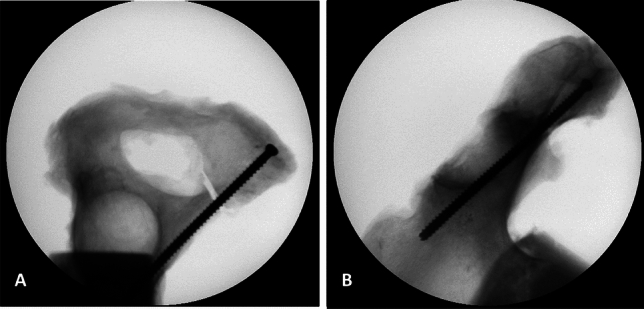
X-ray of superior ramus fracture stabilized with a 6.5 mm cannulated ramus screw; A Inlet-view; B Ala-view
